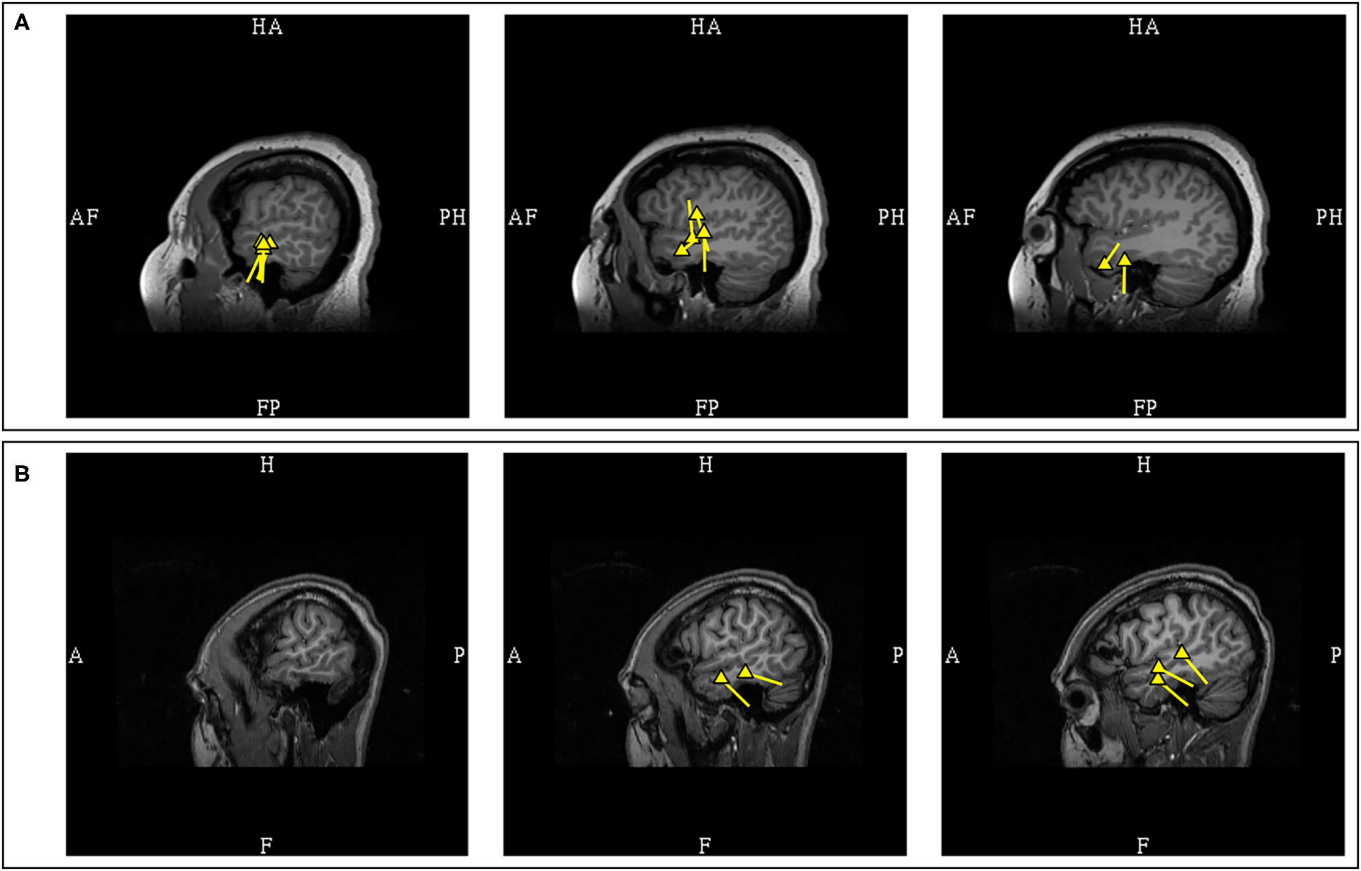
MEG-MRI coregistration summary in two patients with temporal dipoles. (A) Frequent anterior temporal dipoles were suggestive of anterior temporal source. Imaging review revealed temporal tip encephalocele. (B) Temporal dipoles broadly distributed over anterior and posterior temporal region provides comparatively limited suggestion of seizure onset in the absence of a lateral temporal lesion.
